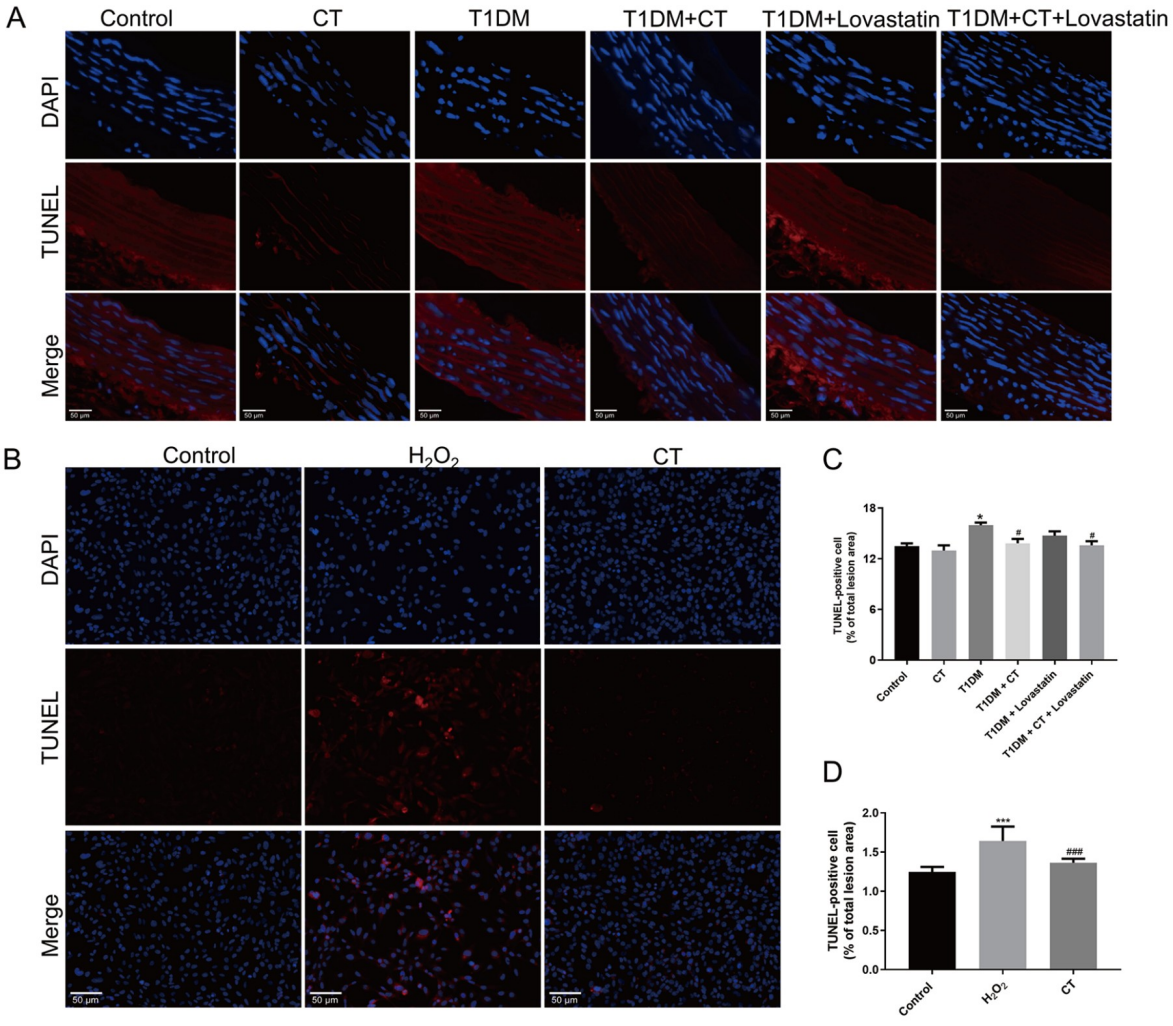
Figure 3 CT inhibits apoptosis of endothelial cells
